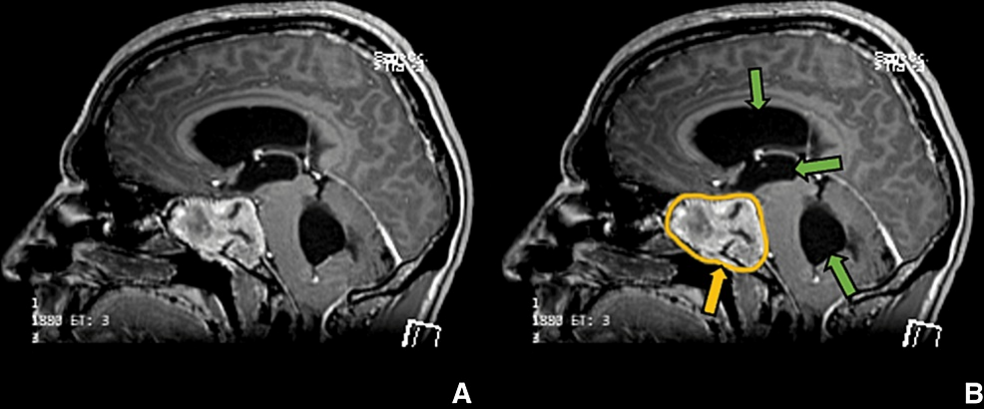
Preoperative imaging for patient 1. Contrast-enhanced T1-weighted sagittal image showing sellar, suprasellar lesion involving clivus and sphenoid sinus. (A) Without ROI and (B) with ROI. The green arrows show hydrocephalus of the lateral, third, and fourth ventricles.
